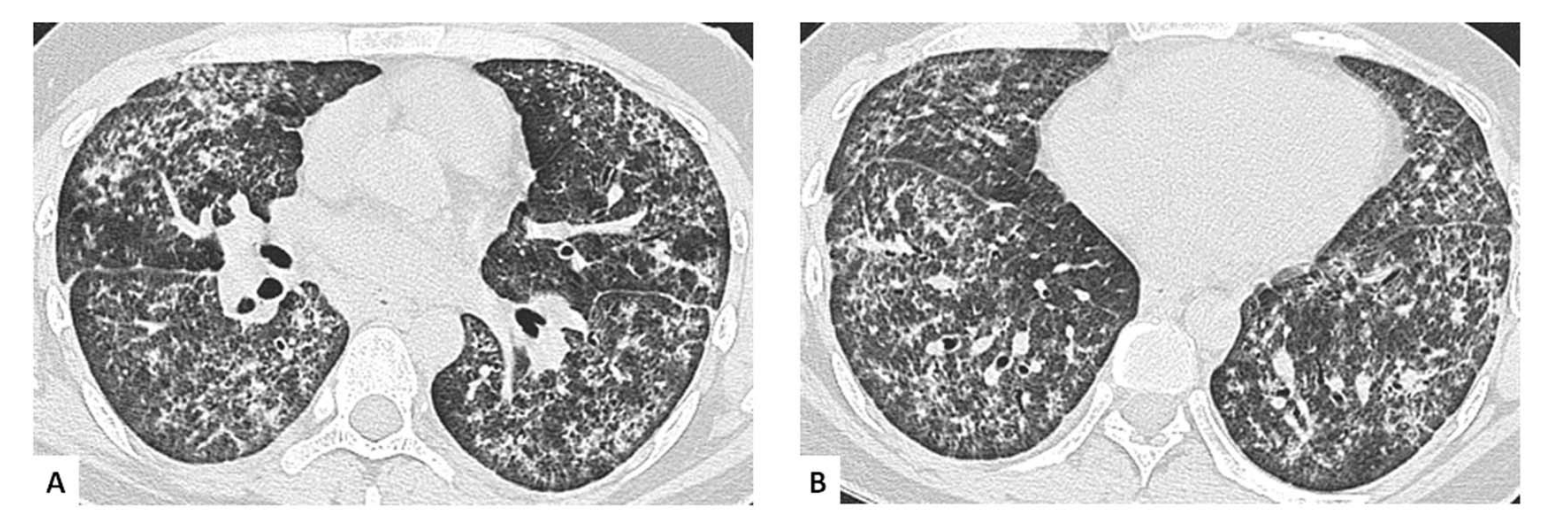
High resolution thoracic CT at time of diagnosis. High resolution CT sections at the level of a) the hila and b) the lung bases demonstrate widespread, bilateral ground glass change, multifocal patches of consolidation and evidence of fibrosis with fine reticulation and traction bronchiectasis.
